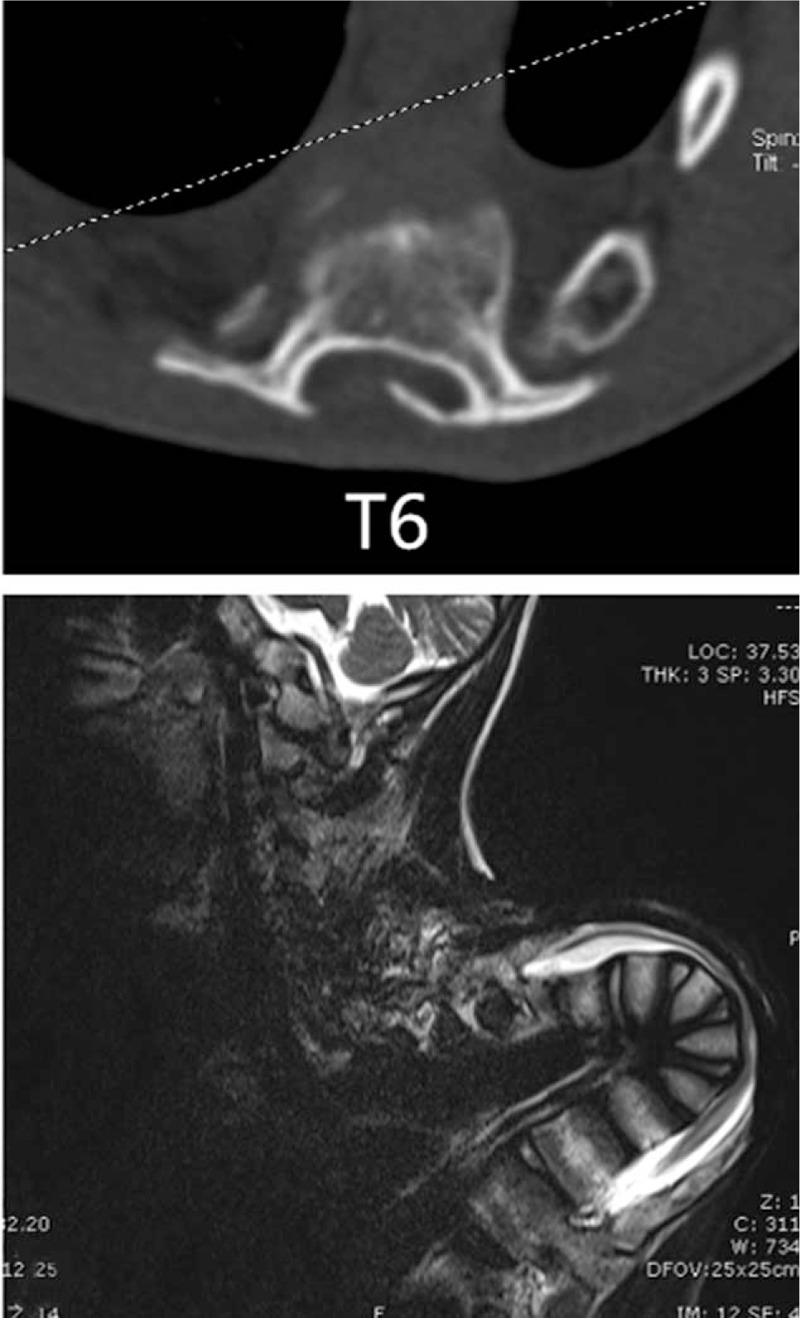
Magnetic resonance imaging (T2-weighted) and CT scans of the spinal canal revealing spinal cord compression at the apex of the kyphosis in the thoracic spine (T6). CT = computed tomography.
